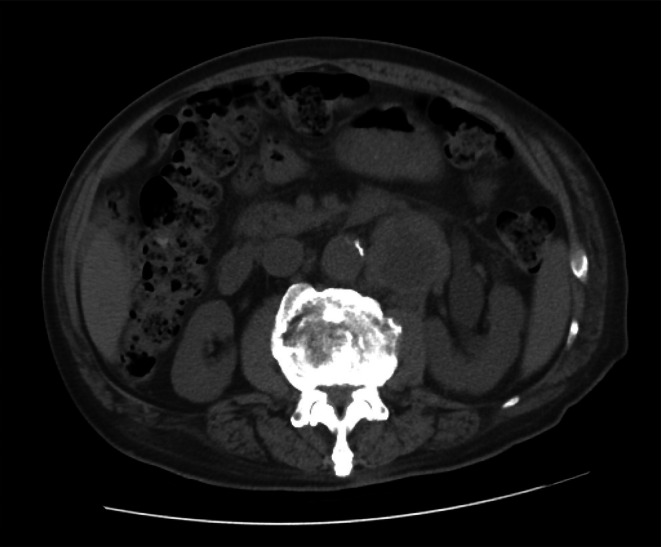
Preoperative CT‐scan image showing para‐aortic lymphadenopathy and left hydronephrosis.
